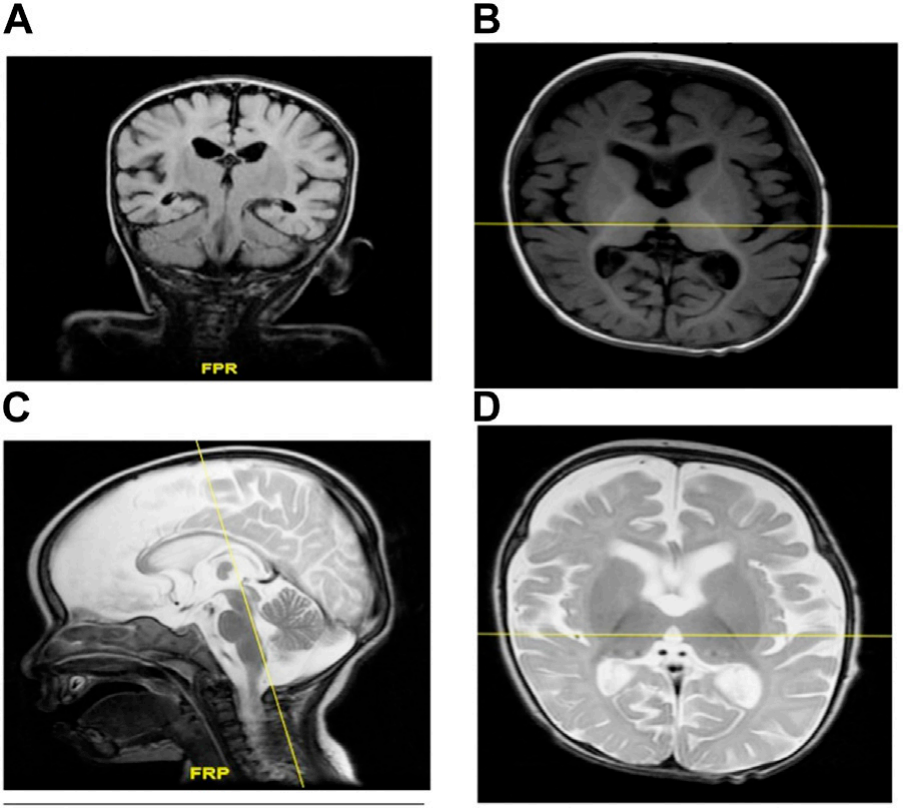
(A–D) MRI of individual II-1 revealed delayed white matter myelination, suggesting that the process of myelin formation in the white matter of the brain has not progressed at the expected rate for an individual of that person’s age. Additionally, the MRI revealed an underdeveloped corpus callosum. The corpus callosum is a vital structure that connects the left and right hemispheres of the brain, allowing for the communication and transfer of information between the two sides. An underdeveloped corpus callosum can lead to impaired communication and integration of information between the brain’s hemispheres, potentially affecting various cognitive functions, motor coordination, and sensory processing.
